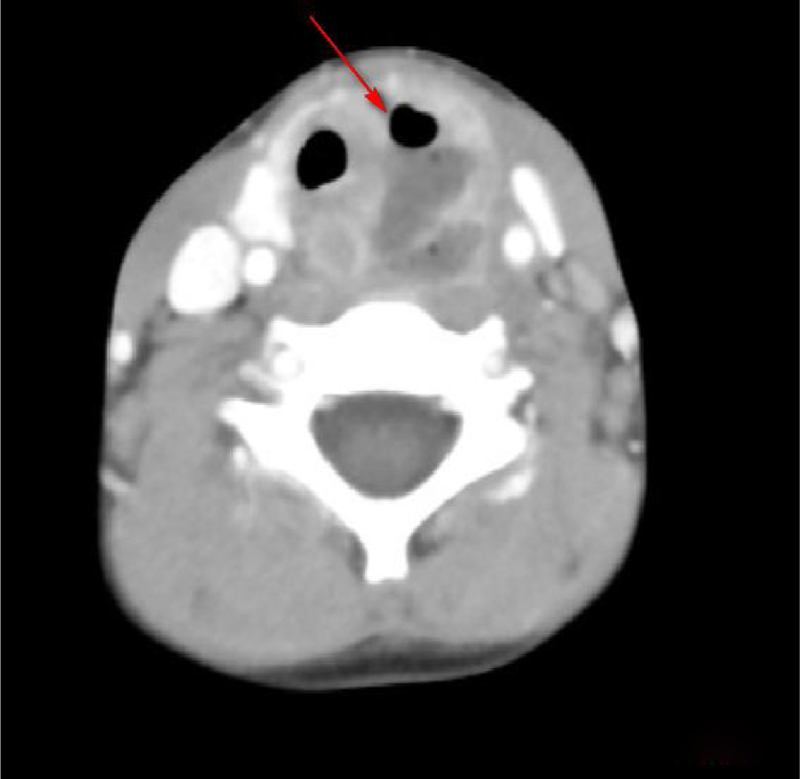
Enhanced CT: left peri-thyroid abscess and gas shadow.
